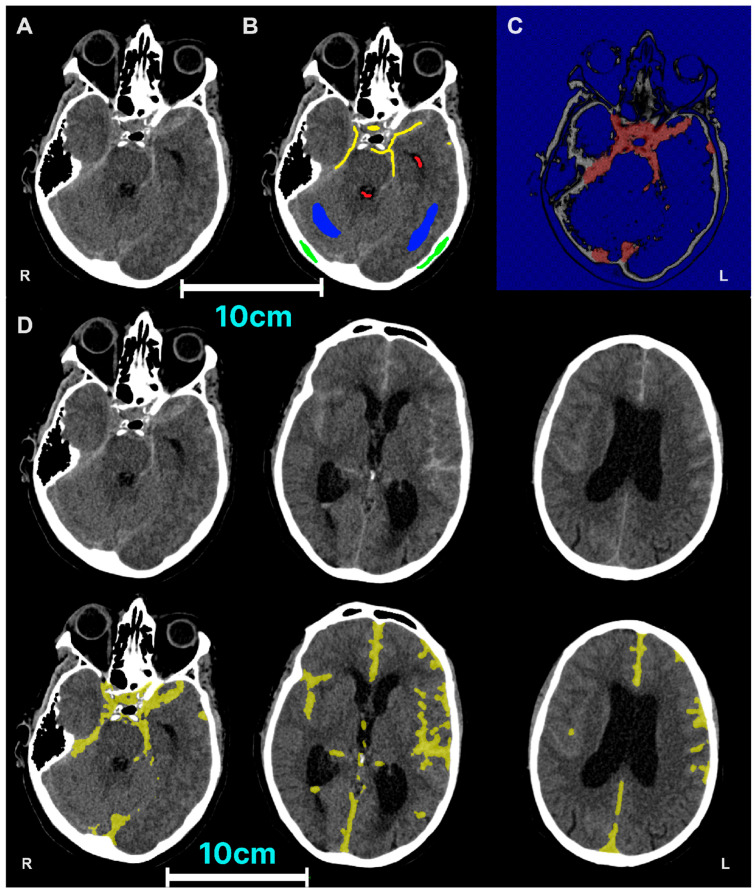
Semi-automated segmentation of SAH. (A) Original CT. (B) Manual labelling of different brain tissues, i.e., classification. Red represents cerebrospinal fluid, green represents bone, blue represents brain parenchyma and yellow represents subarachnoid haemorrhage. (C) Evolution of contours. (D) Final segmentation at different levels after manual inspection and editing. (Single column with colour in print).
